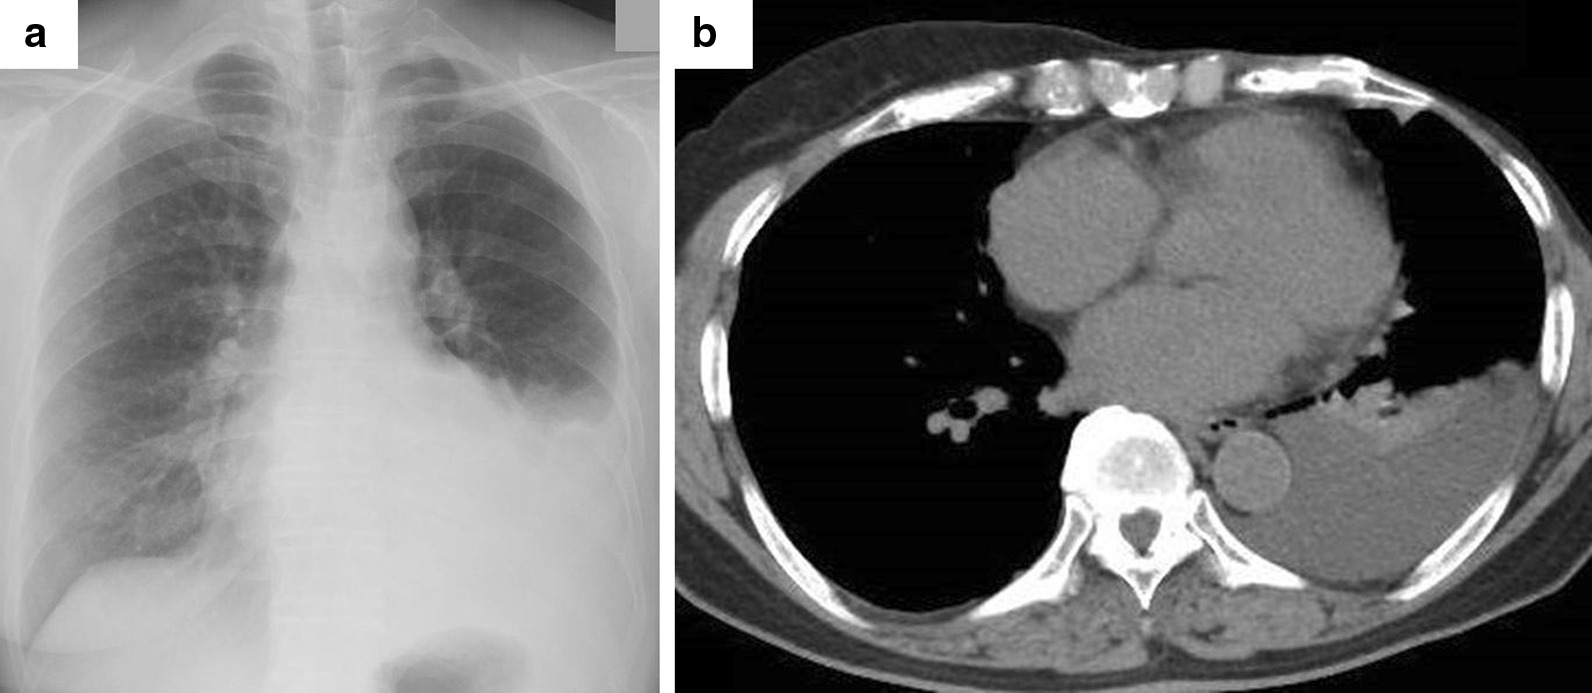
Radiological findings. Chest X-ray at admission showed pleural effusion (a). Chest CT scan showed no thickening, calcification, or nodules in the left pleura. No tumour nodules were suspected in the lungs (b)
